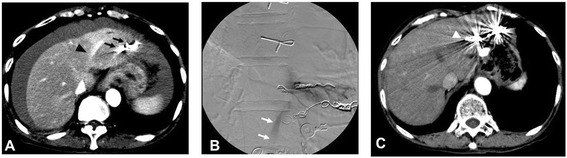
Contrast-enhanced computed tomography and angiography. (A) Contrast-enhanced computed tomography to diagnose bleeding. Remarkable leakage of contrast medium from the left inferior phrenic vein was seen (black arrowhead). There was excessive blood around the liver. There were coil embolizations of shunts (black arrows). (B) Angiography revealed leakage of contrast medium (white arrows) from the left inferior phrenic vein. (C) Contrast-enhanced computed tomography 2 months later. There were no ascites around the liver. There were coils for hemostasis (white arrowhead).
